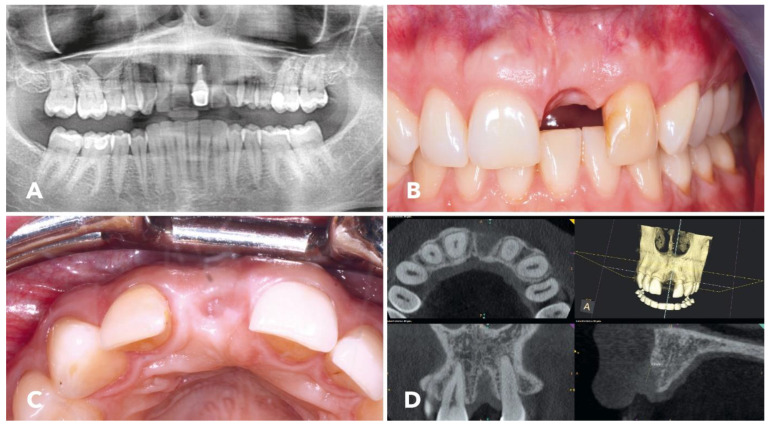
(A) Preoperative panoramic radiograph showing a periradicular translucent lesion on tooth 21; clinically, a buccal fistula was present. (B) Clinical situation of the patient 4 months after the extraction of tooth 21; the distal papilla had receded and the buccal wall 21 was atrophic; the soft tissue situation was sound and free of any inflammation; a wide zone of keratinized gingiva was visible. (C) Clinical situation in the palatinal view; a slight lack of buccal bone was evident; no signs of inflammation. (D) The cone beam CT showed the transverse bone defect, which corresponded to a class II defect according to the ITI classification.
